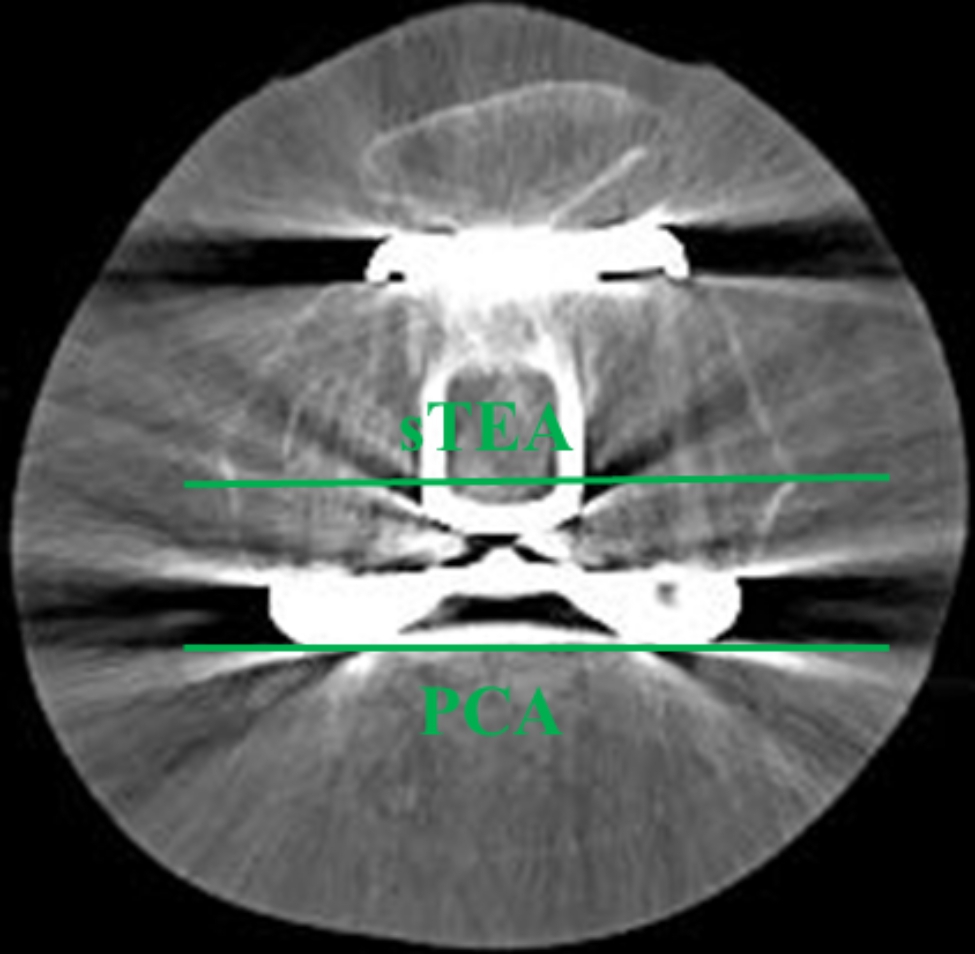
Component position angle on CT. The FCR was determined as the angle between the sTEA and PCA ^*^CT, computer tomography; FCR, femoral component rotation; sTEA, surgical transepicondylar axis; PCA, posterior condylar axis
